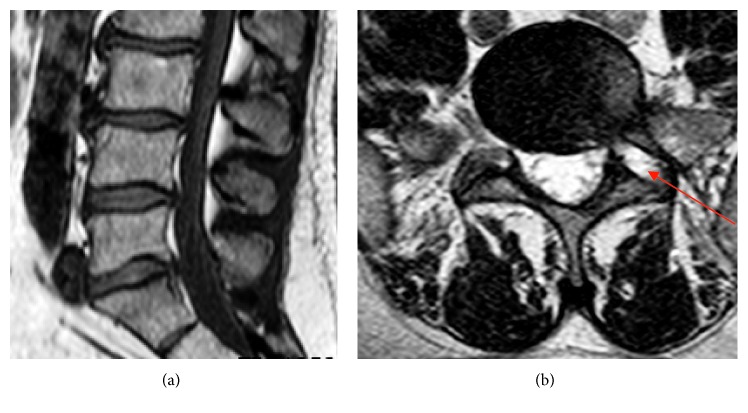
Postoperative lumbar MRI with completely removed sequester and bone hole through the pedicle.
